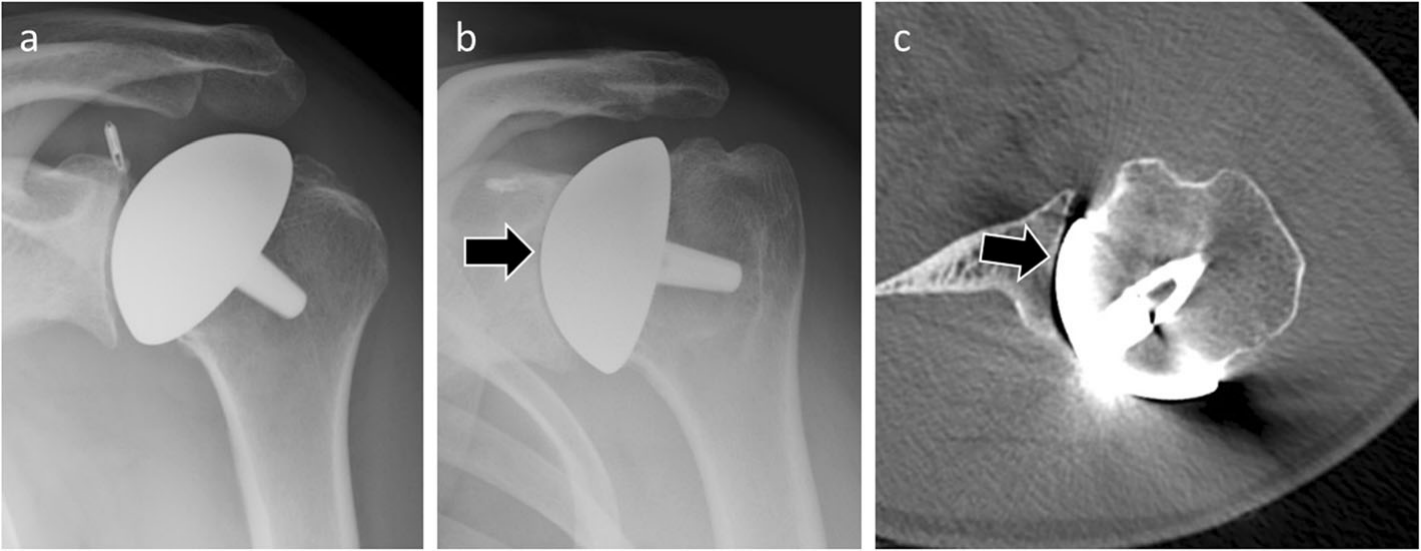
a Anteroposterior radiograph of a 36-year-old man with humeral head resurfacing arthroplasty. Anteroposterior radiograph (b) and axial CT image (c) in the same patient as a, 7 years later, show native glenoid wear manifested by narrowing of the glenohumeral spacing (arrows)
